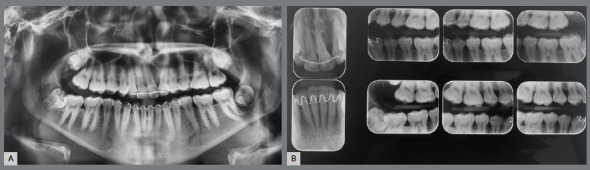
Final panoramic radiograph (A) and final periapical radiographs (B).
